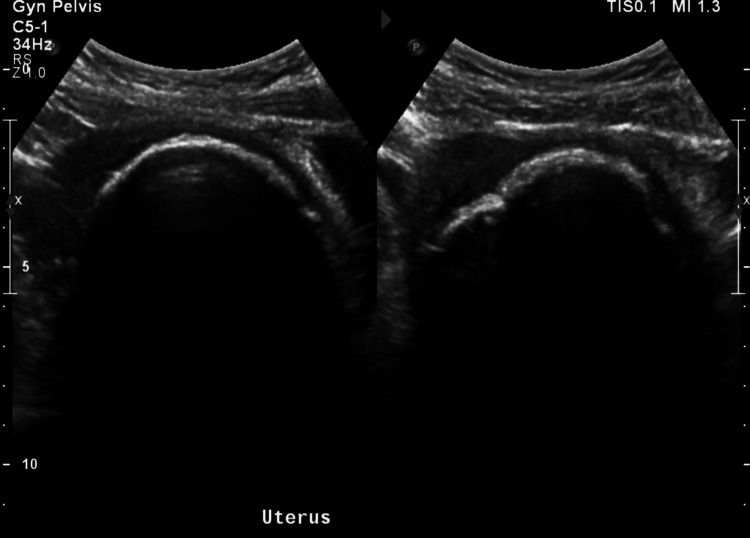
Pelvic US: sagittal view (left) and transverse view (right) US: ultrasound
